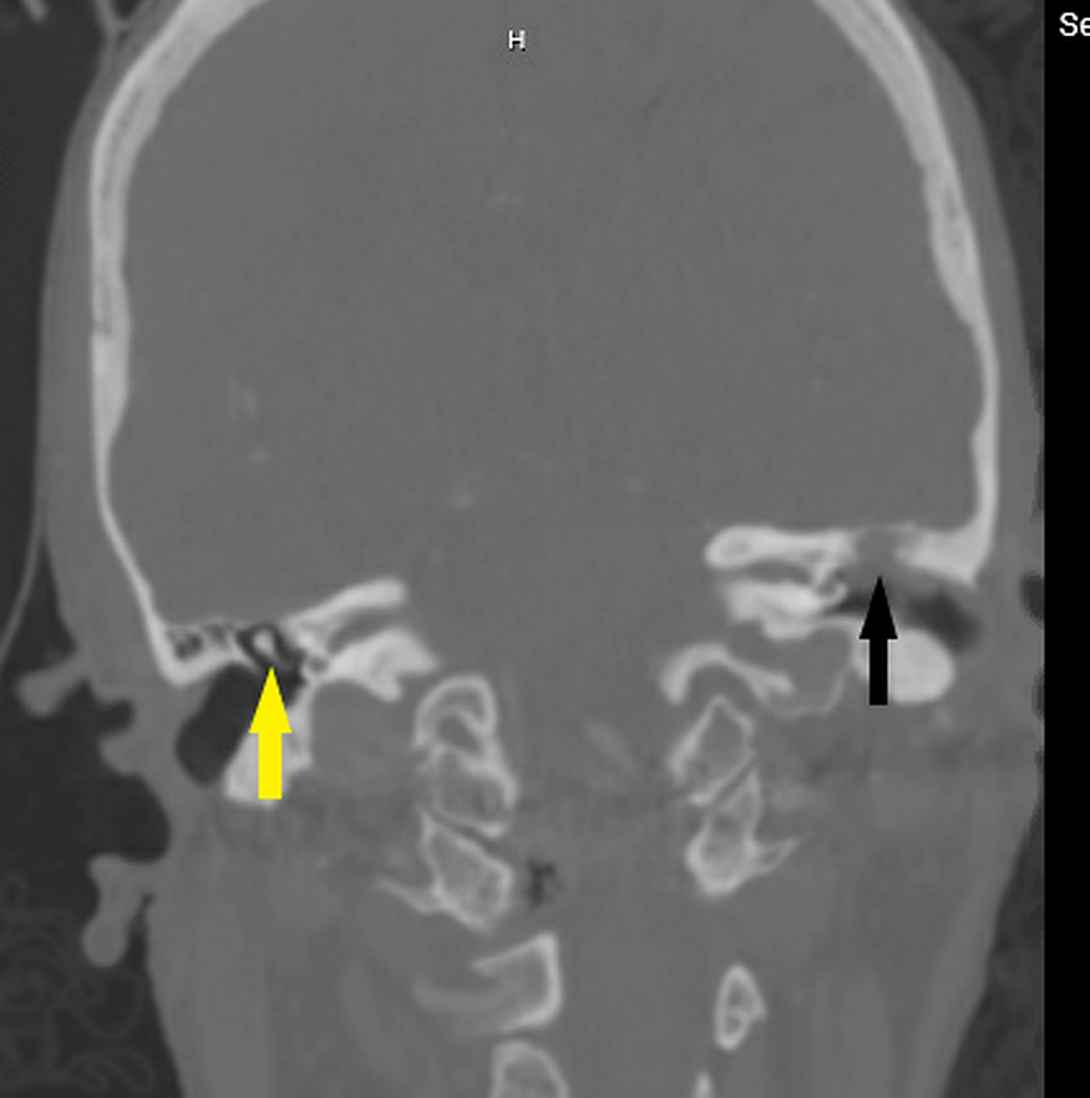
CTA of the patient The presence of cholesteatoma (black arrow) can be noted in the left middle ear cavity, visible as an opacification. Compare with normal-appearing malleus (yellow arrow) and the middle ear on the right side CTA: computed tomography angiography
